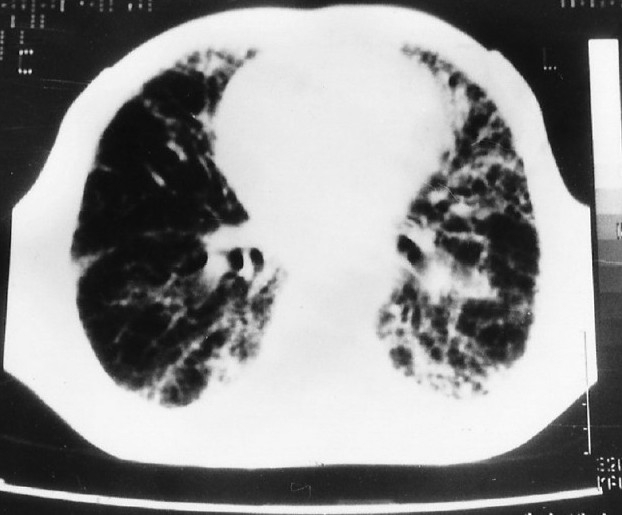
CT scan thorax showing interstitial lung disease pattern
